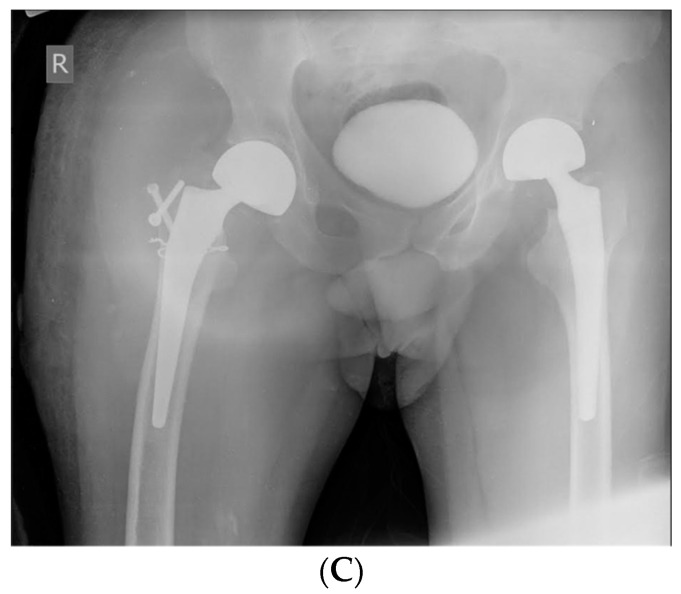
(A) Case 1—X-ray: Right femoral neck fracture—before surgery. (B) Case 1—X-ray: Left femoral neck fracture—before surgery. (C) Case 1—X-ray: Bilateral simultaneous hip arthroplasty, the right hip joint was complicated with pertrochanteric fracture and needed additional osteosynthesis with screws.
